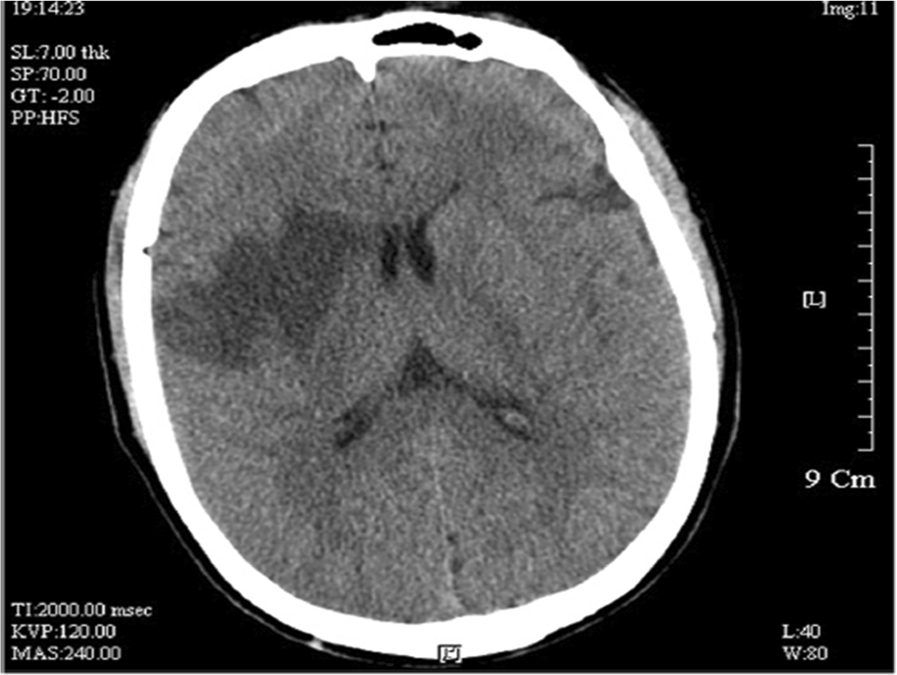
CT showed hypodense lesions in the right basal ganglia.
